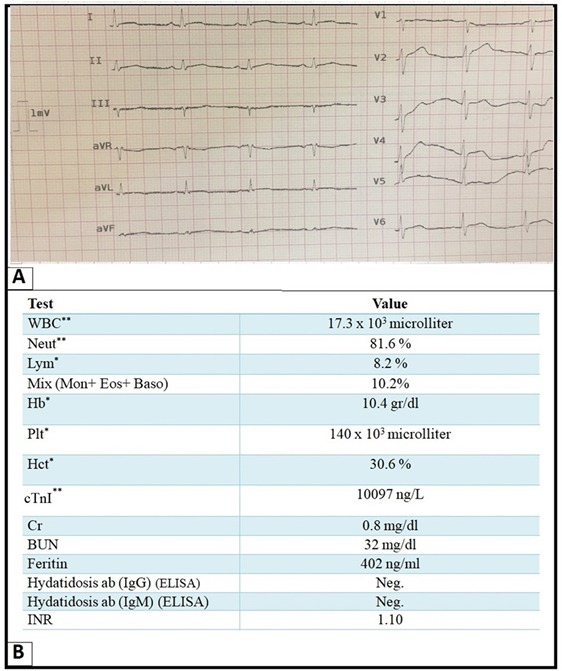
Transthoracic echocardiography (TTE) demonstrated a well-defined cyst (2×2.1 cm) with thickened wall in interventricular septum (IVS, at its mid-portion). Left ventricle (LV) was hypertrophied (septal thickness: 1.3 cm; posterior wall thickness: 1.44 cm) and its systolic function was reduced (LVEF #45%). Moreover, large pericardial effusion, with significant hemodynamic compromise (collapse of the right atrium (RA) and the RV) was evident (Figure 2 A, B & C). Pulmonary arterial pressure was normal.
